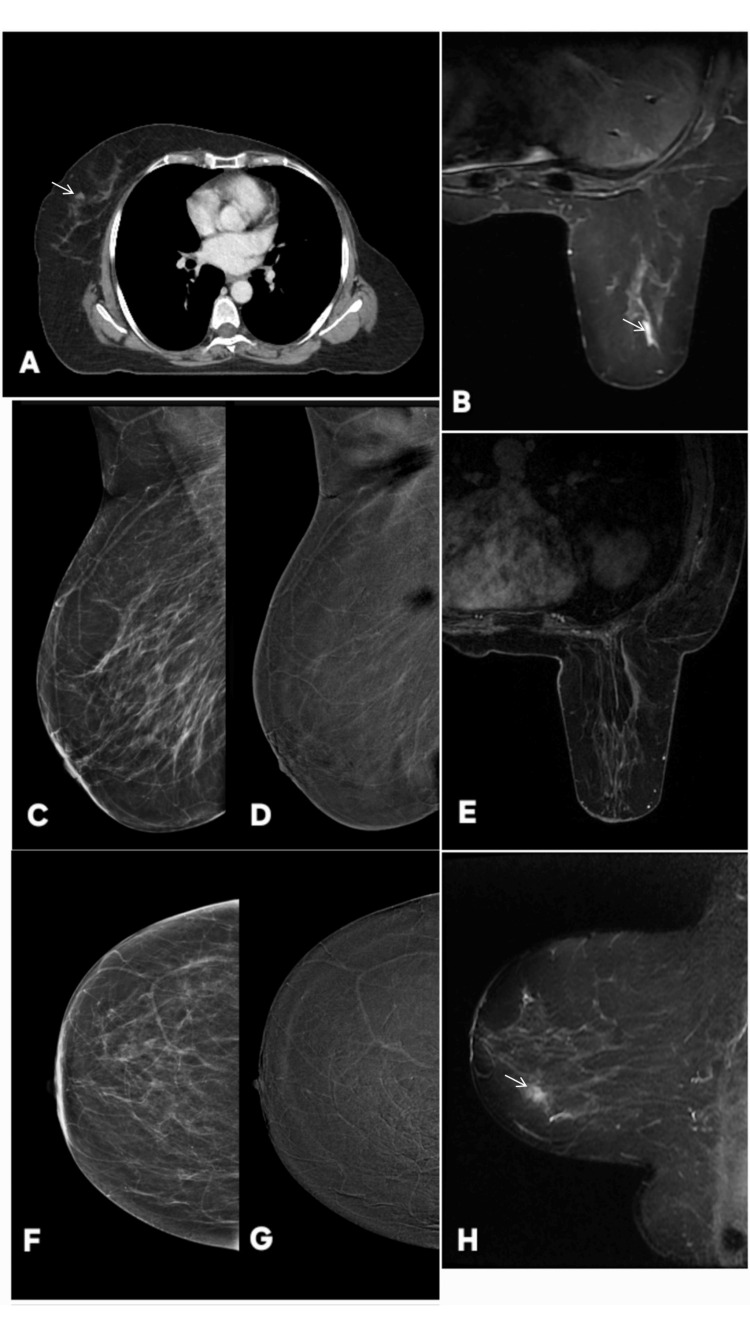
Contrast-enhanced mammography (CEM) correctly downgrading a suspicious focal enhancement in a 60-year-old surveillance female patient with treated left-breast invasive lobular carcinoma. The abnormality is annotated by white arrows in all images. A: Routine follow-up contrast-enhanced CT (axial) at level of right breast shows ill-defined focal area of parenchymal enhancement (white arrow). Although this could represent a summation artifact, an evolving focal abnormality could not be excluded, prompting further evaluation with breast MRI. Unfortunately no previous CT is available for comparison. B & H: Contrast-enhanced T1-weighted MRI of the right breast (axial in B; sagittal in H) demonstrates a linear enhancing focus in the same region, raising concern for a potentially enhancing duct or subtle non-mass enhancement. The configuration remained suspicious, necessitating correlation with mammography and CEM (BI-RADS 4). E: Corresponding T2-weighted axial MRI shows no discrete abnormality at the site of concern, resulting in discordant multiparametric MRI features and maintaining diagnostic uncertainty. C & F: Digital mammography (MLO and CC) demonstrates no focal mass, architectural distortion, or asymmetry at the site marked on CT/MRI. Parenchymal pattern remains stable compared with prior examinations (BI-RADS 2). D & G: CEM (MLO and CC) shows no areas of abnormal contrast uptake, excluding an enhancing mass or non-mass lesion. No focal parenchymal enhancement is seen on CEM subtraction images, and breast vascularity is normal (BI-RADS 2). Targeted ultrasound (not shown) also demonstrated no correlating abnormality. Despite suspicious focal enhancement on CT and equivocal linear enhancement on MRI, CEM demonstrated complete absence of enhancing parenchymal abnormality, allowing confident downgrading of the finding. Ultrasound after six months revealed normal breast. This underscores the value of CEM as a highly effective problem-solving tool, particularly when MRI findings are subtle, discordant, or inconclusive, and highlights its usefulness in surveillance imaging of patients with history of lobular carcinoma. MLO: mediolateral oblique; CC: craniocaudal; BI-RADS: Breast Imaging Reporting and Data System
